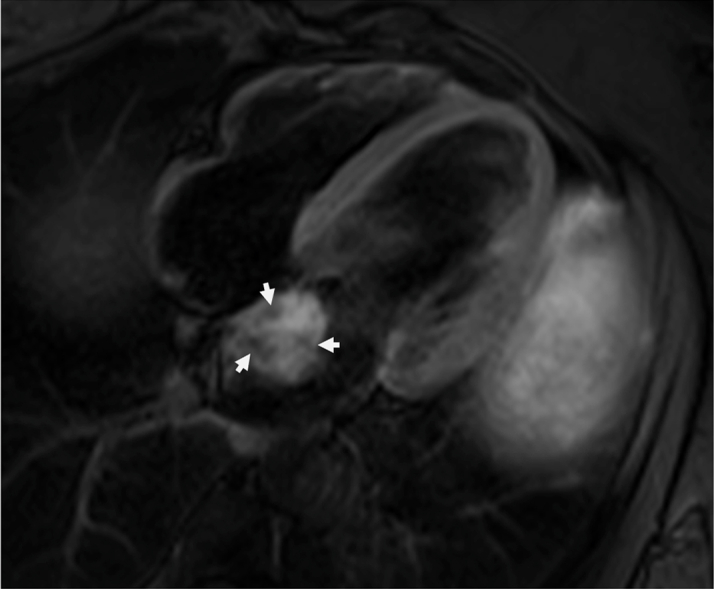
T2-Weighted Cardiac Magnetic Resonance The mass is hyperintense to the myocardium on T2-weighted images. The thrombus is labeled with open arrows, which appears hypointense on T2 imaging compared with the rest of the myxoma.
